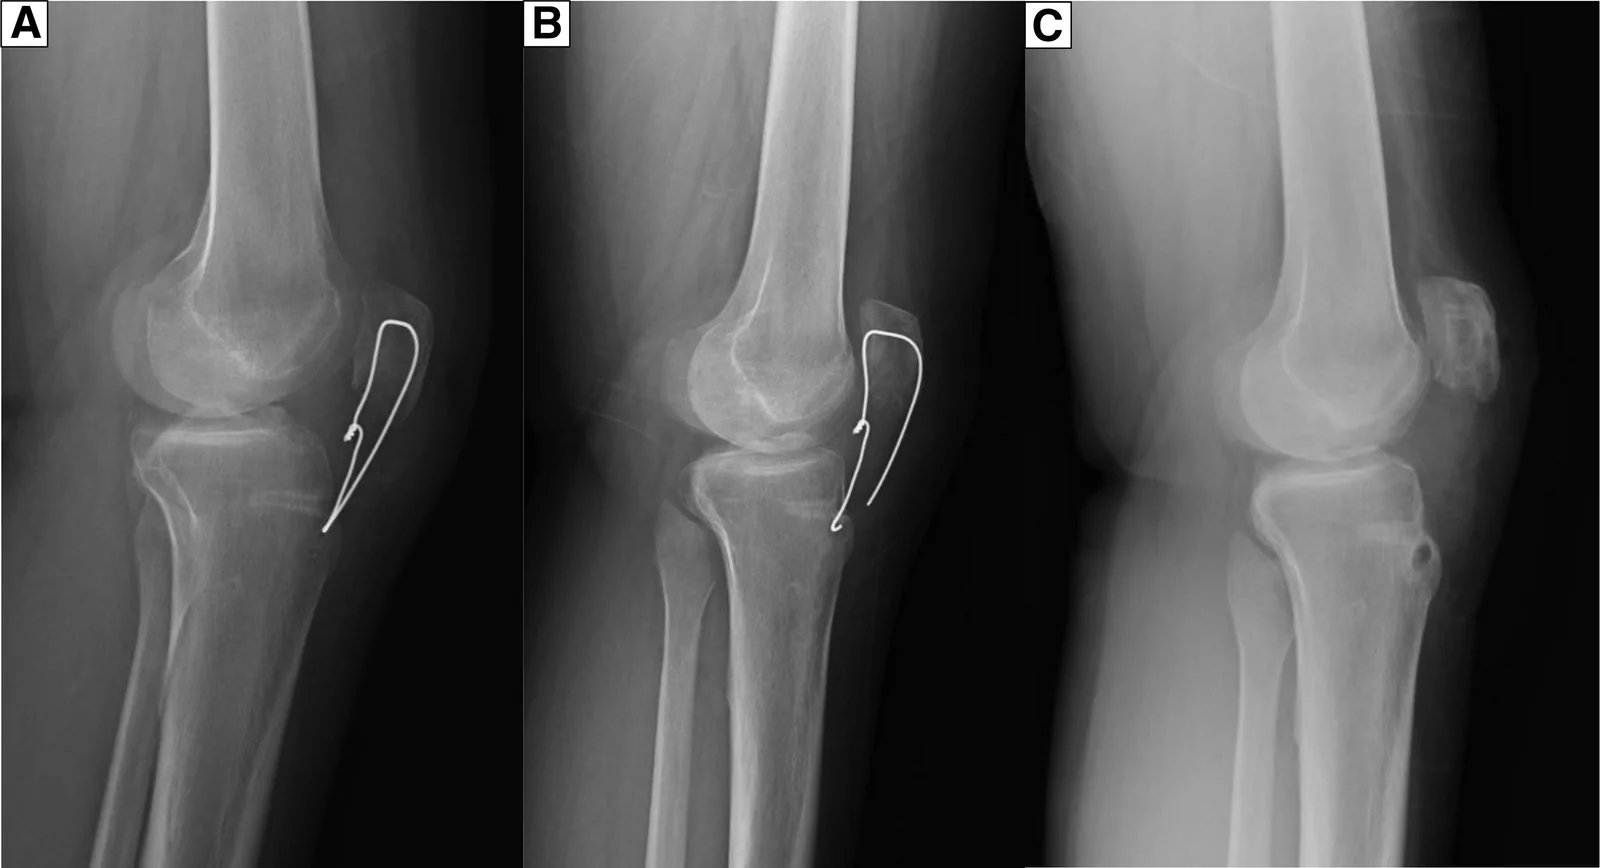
Serial postoperative lateral radiographs. (A) Immediate postoperative lateral radiograph (postoperative day 0) demonstrating an Insall–Salvati ratio of 0.80 following intraoperative tensioning. (B) Radiograph obtained 6 months postoperatively incidentally demonstrating breakage of the cerclage wire. (C) Radiograph obtained 12 months postoperatively after hardware removal demonstrating maintained patellar height with a balanced Insall–Salvati ratio of 1.08.
